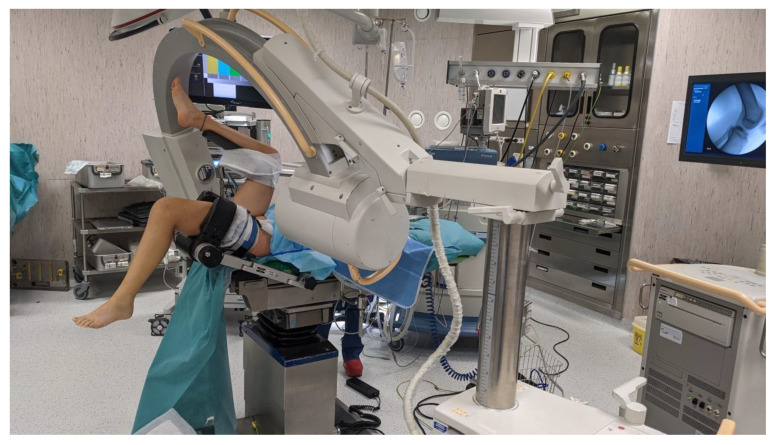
Operating theatre setup: the patient is in a supine position with the affected leg immobilized in a leg holder and the opposite leg held with a gynecologic leg holder to grant adequate workspace. C-arm fluoroscopy is set to radiographically guide fracture reduction and osteosynthesis with Kirschner wires.
